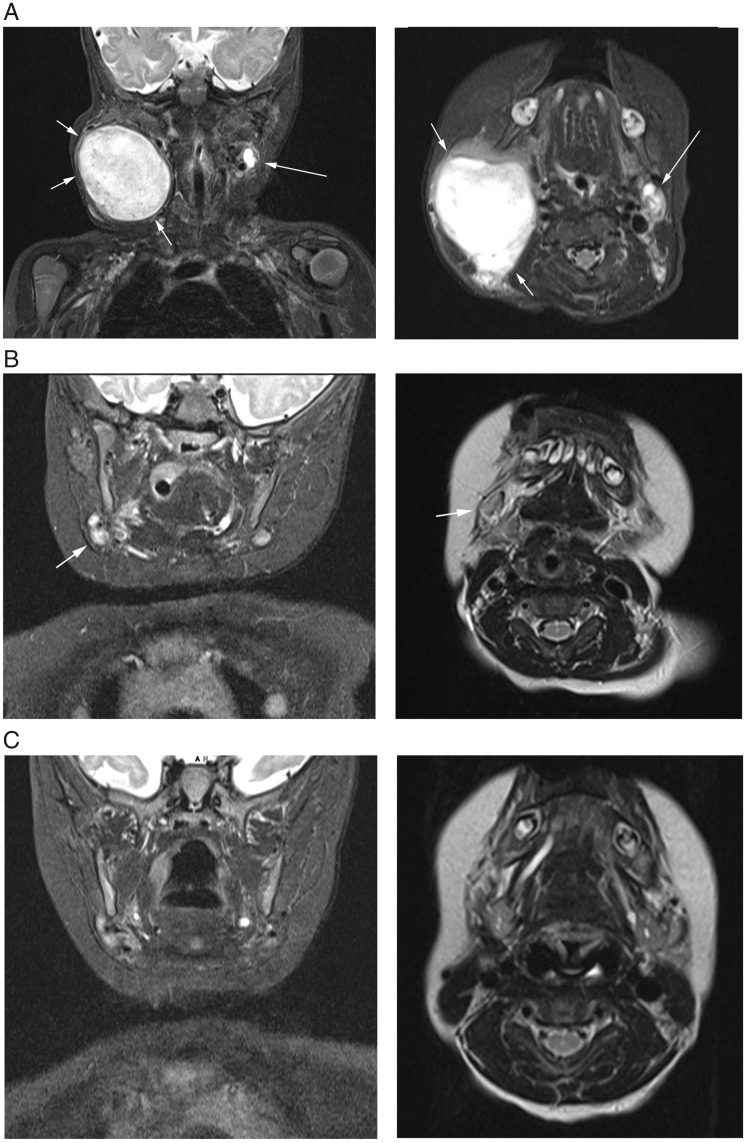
Visualisation of the largest diameters of the right cervical lesion upon coronal and planar magnetic resonance imaging (A) at screening, (B) on day 56 of treatment and (C) on day 112 of treatment.
